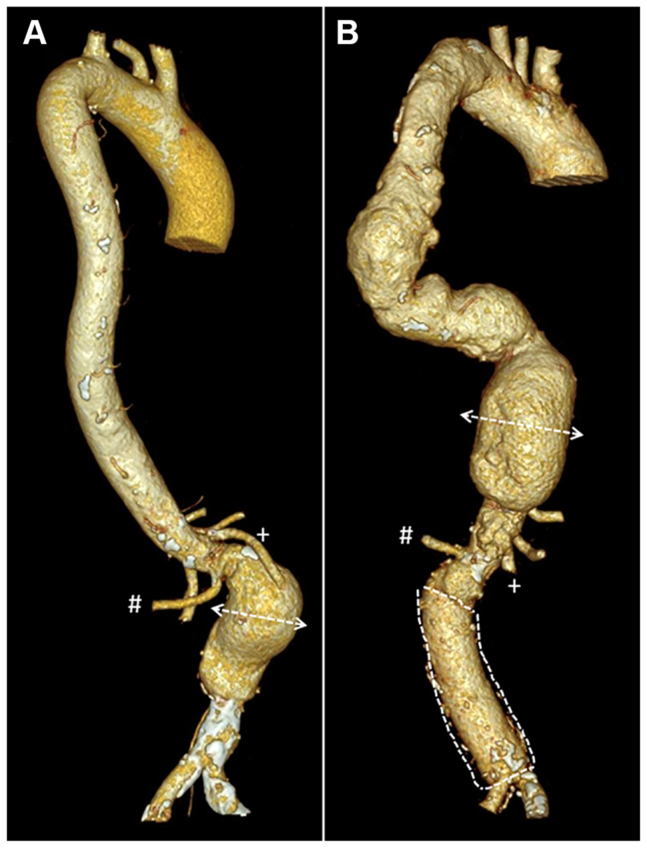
Three-dimensional computed tomography angiography reconstruction from representative patients (*MIP*, maximum intensity projection: thrombus not visible). (A) Dorsal view for a typical patient with juxtarenal AAA (male, 76 years old; *dotted arrow*, maximum axis corrected diameter 65 mm) before open repair (#, left renal artery; +, right renal artery). (B) Dorsal view of a patient with TAAA (female, 67 years old; *dotted arrow*, maximum axis corrected thoracic diameter 65 mm; previous maximum axis corrected abdominal diameter 60 mm) after infrarenal abdominal repair with a 28-mm Dacron tube graft (*dotted line*). Samples were collected from the anterior wall of the abdominal part of the aneurysm around the largest circumference in both cases.
